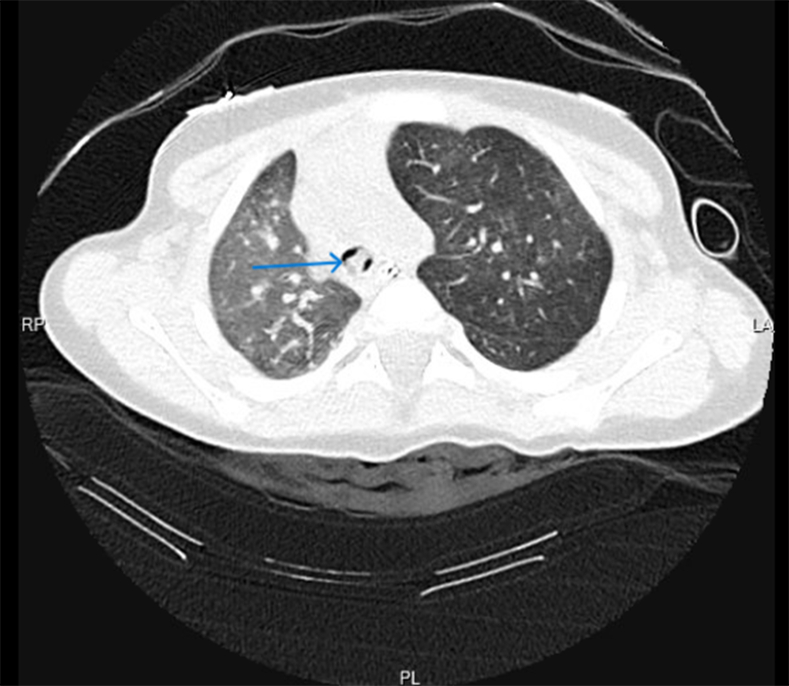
Chest CT scan (cross-sectional view) shows foreign body (blue arrow). CT, computed tomography.
